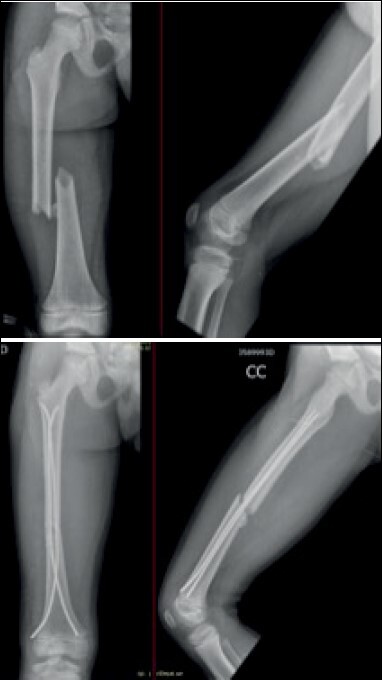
Immediate pre- and postoperative radiography of diaphyseal femur fracture.
